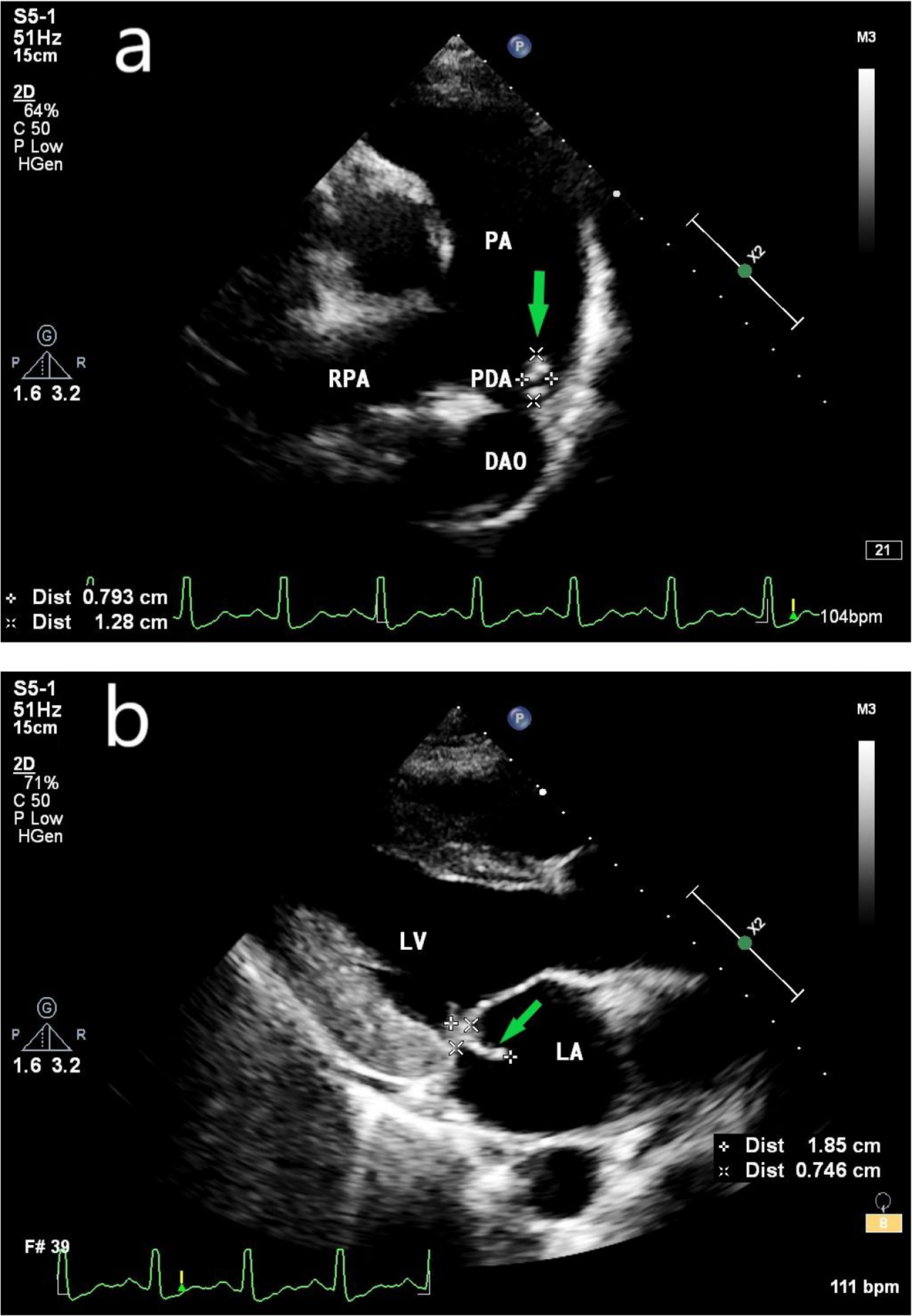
a Echocardiography at 31 weeks of gestation shows that the vegetation has shrunk. b No obvious reduction of mitral valve vegetation. (PA = pulmonary artery; PDA = patent ductus arteriosus; RPA = right pulmonary artery; DAO = descending aorta; green arrow = infective endocarditis vegetative)
